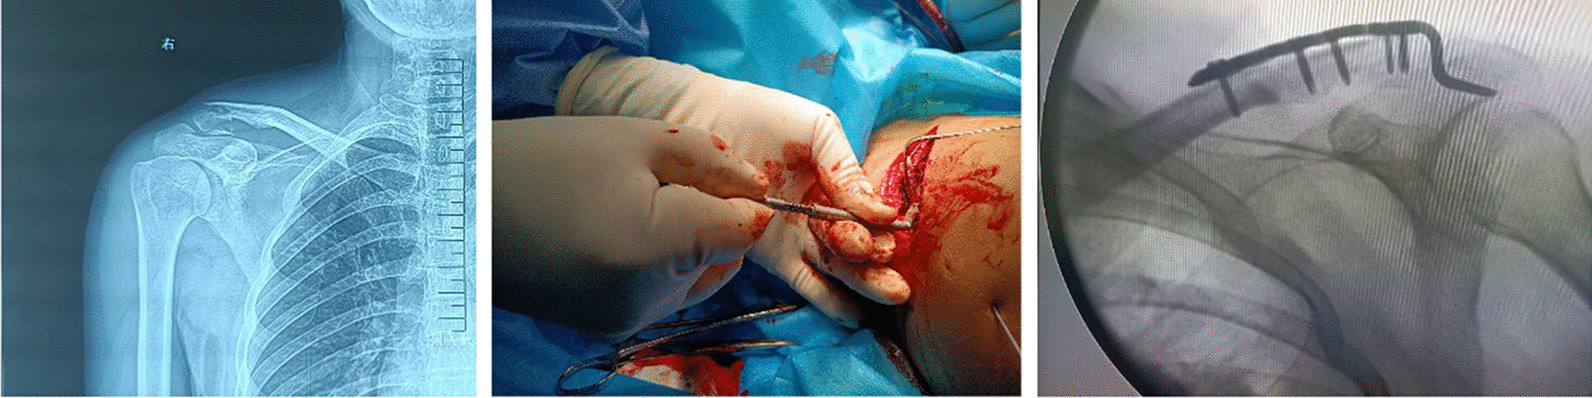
The patient's preoperative X-ray showed that the bone continuity of the right distal clavicle was interrupted; we chose to use the new-type hook plate fixation, which avoided the fracture line well and made the fixation firm
